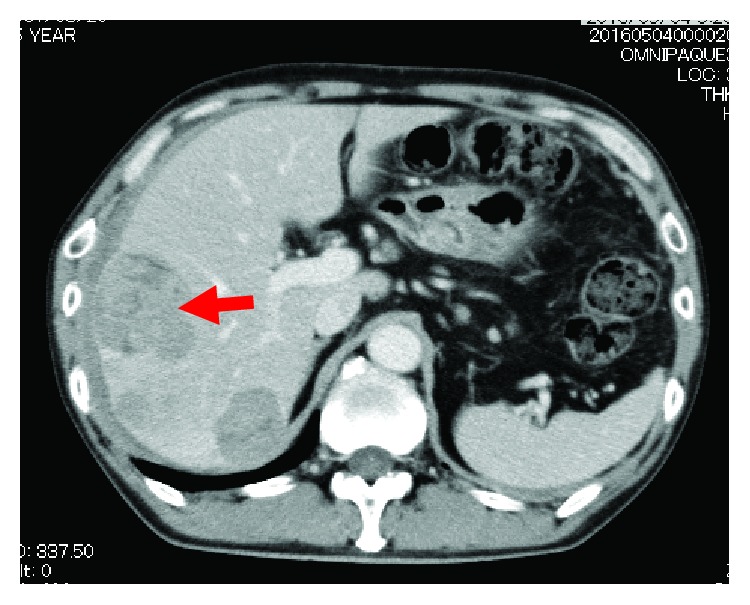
CT scan showing multiple liver metastases suggestive of hepatocellular carcinoma. The red arrow indicates the mass rupturing imminently.
